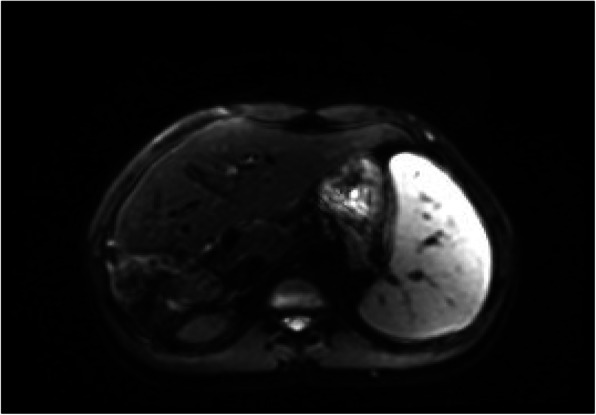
MRI of the abdomen cavity permormed after the operation – numerous calcified lesions and splenomegaly
